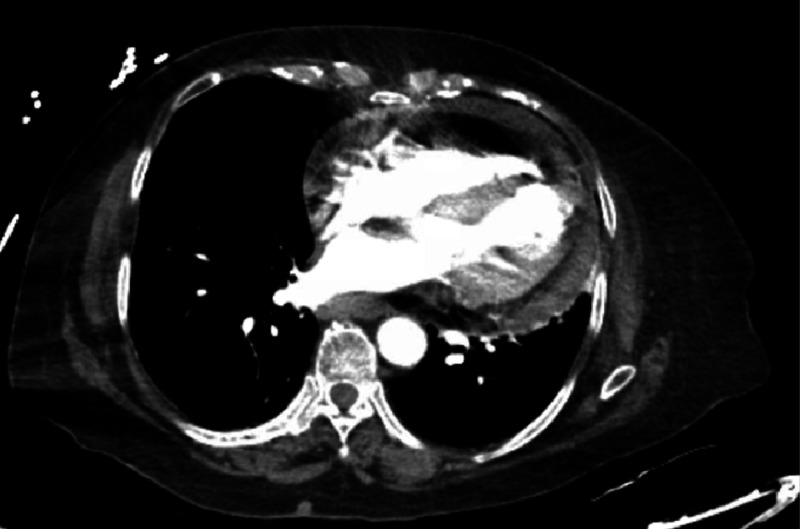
CT chest angiogram showed complex pericardial effusion compatible with hemopericardium.
